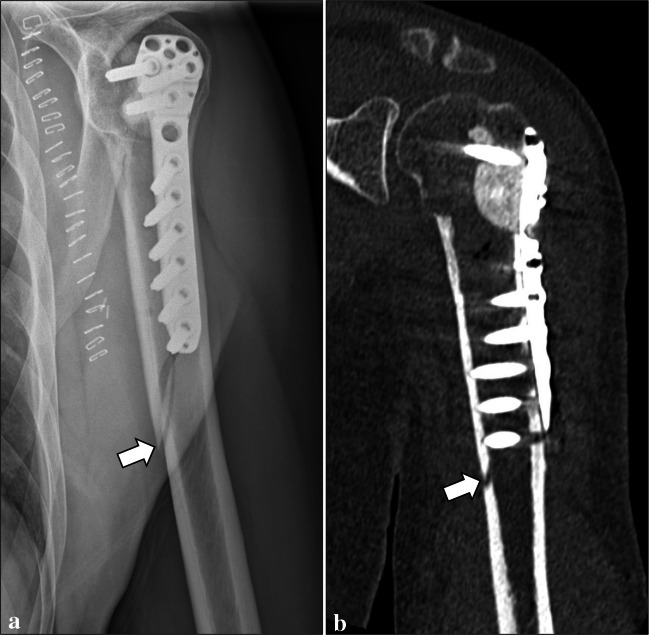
Perioperative bone fracture in a 65-year-old man. A non-displaced fracture of the humeral diaphysis (arrows) was noted after curettage of an ACT of the proximal humerus, bone grafting and internal fixation on radiograph (a) and CT (b)
